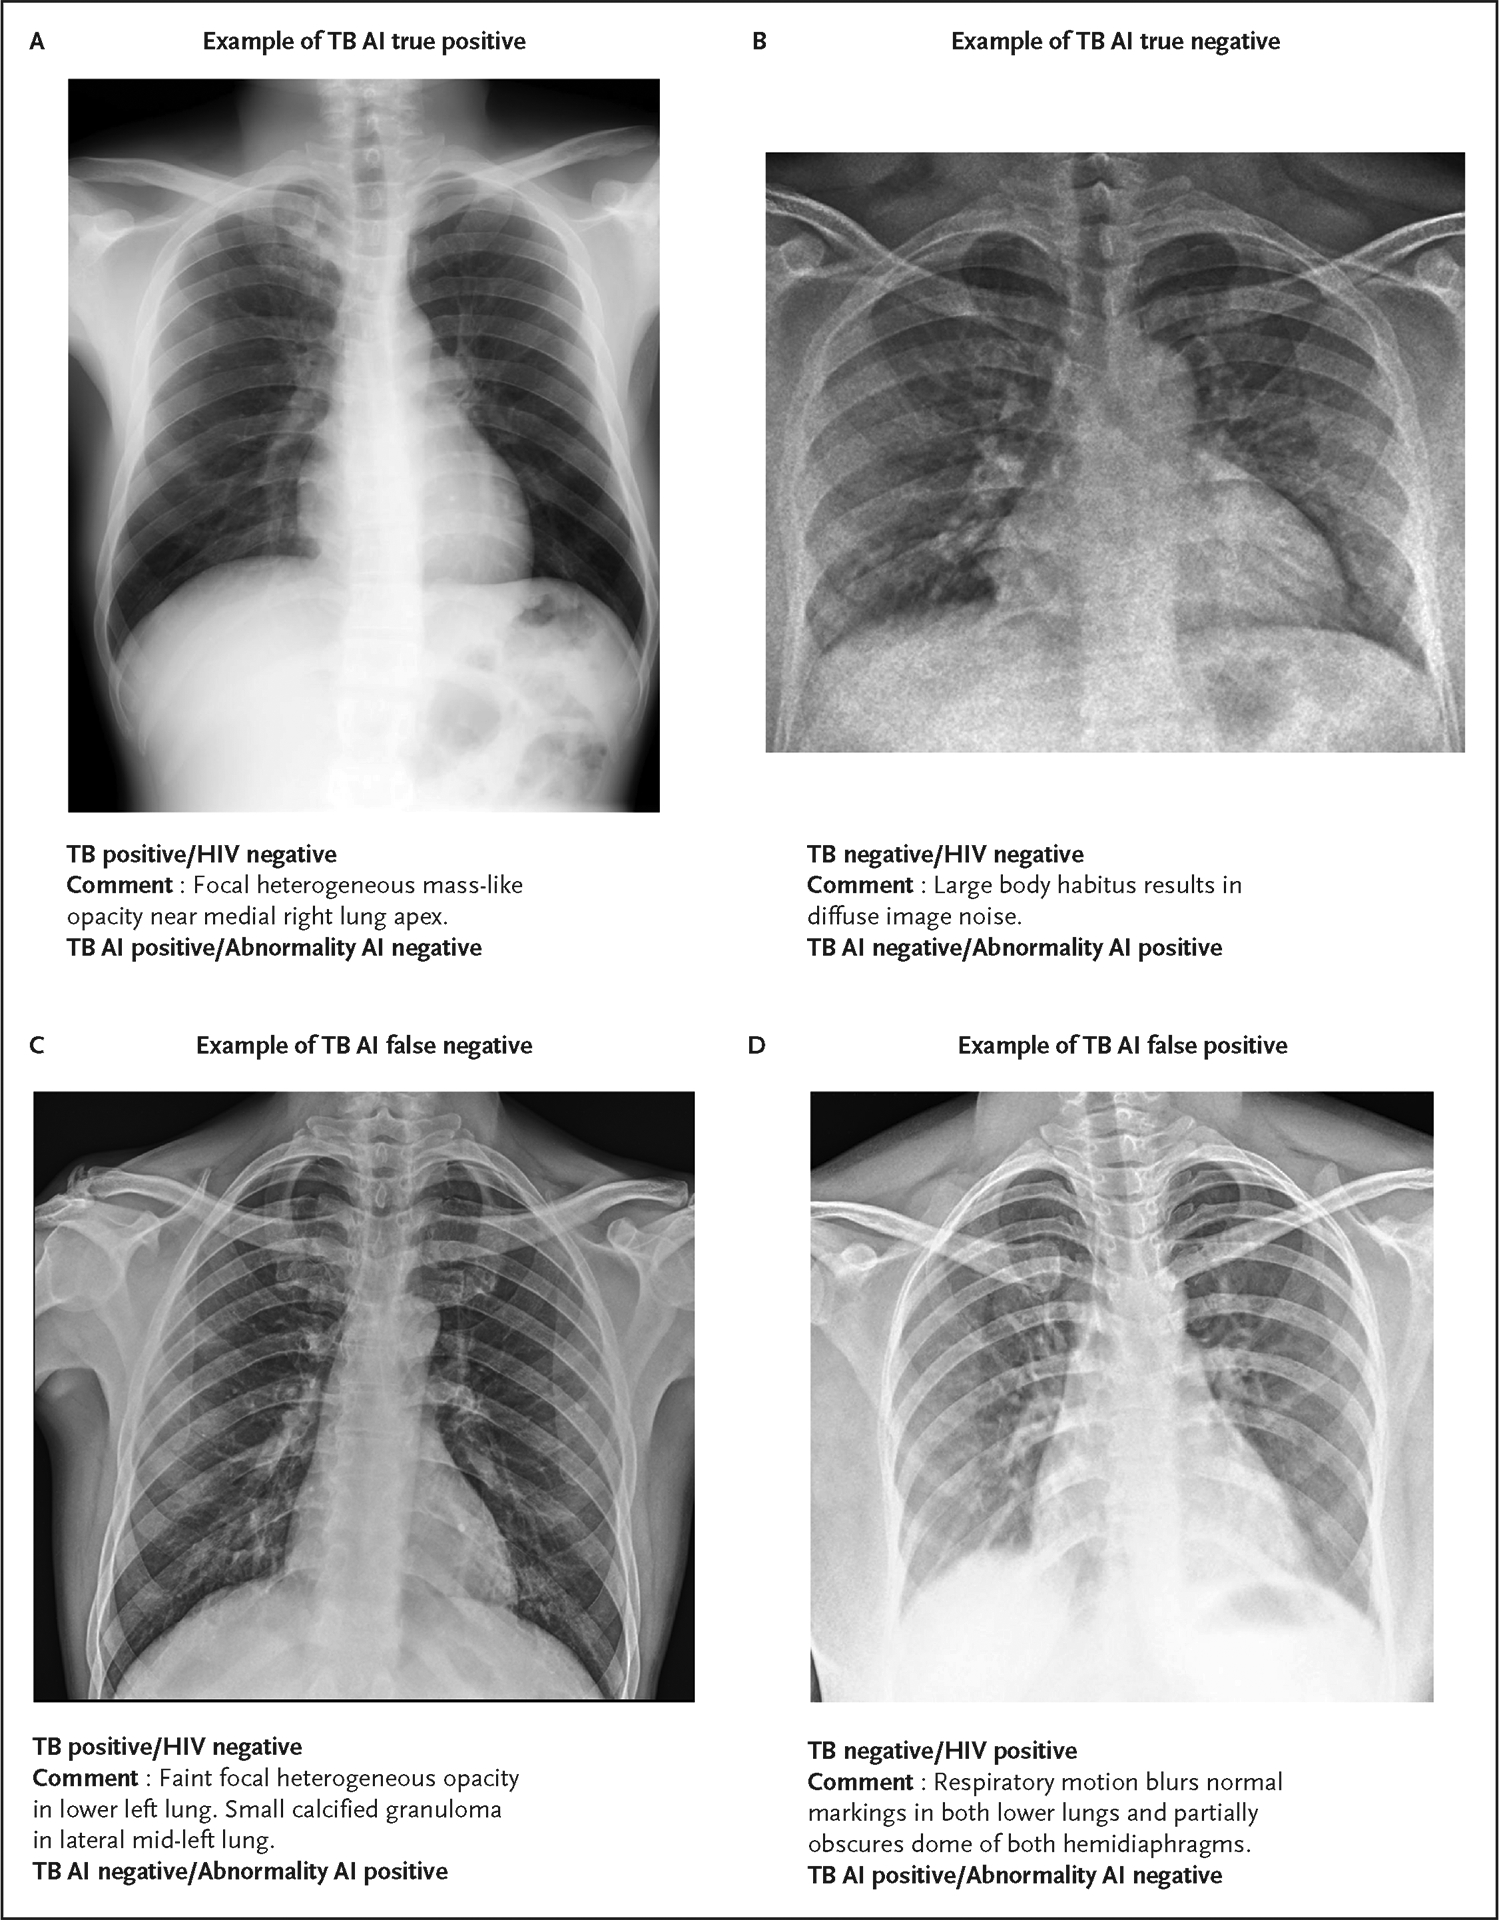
Example Chest X-Rays on Borderline Decision Categories and AI Model Differences. Panel A shows true positive cases where the abnormality AI model is deemed negative, Panel B shows an example of a TB true negative where the abnormality AI was positive, Panel C shows a TB AI false negative with an abnormality AI positive, and Panel D shows a TB AI false positive with an abnormality AI negative. Comments per panel reflect those from an experienced thoracic radiologist. TB denotes tuberculosis.
